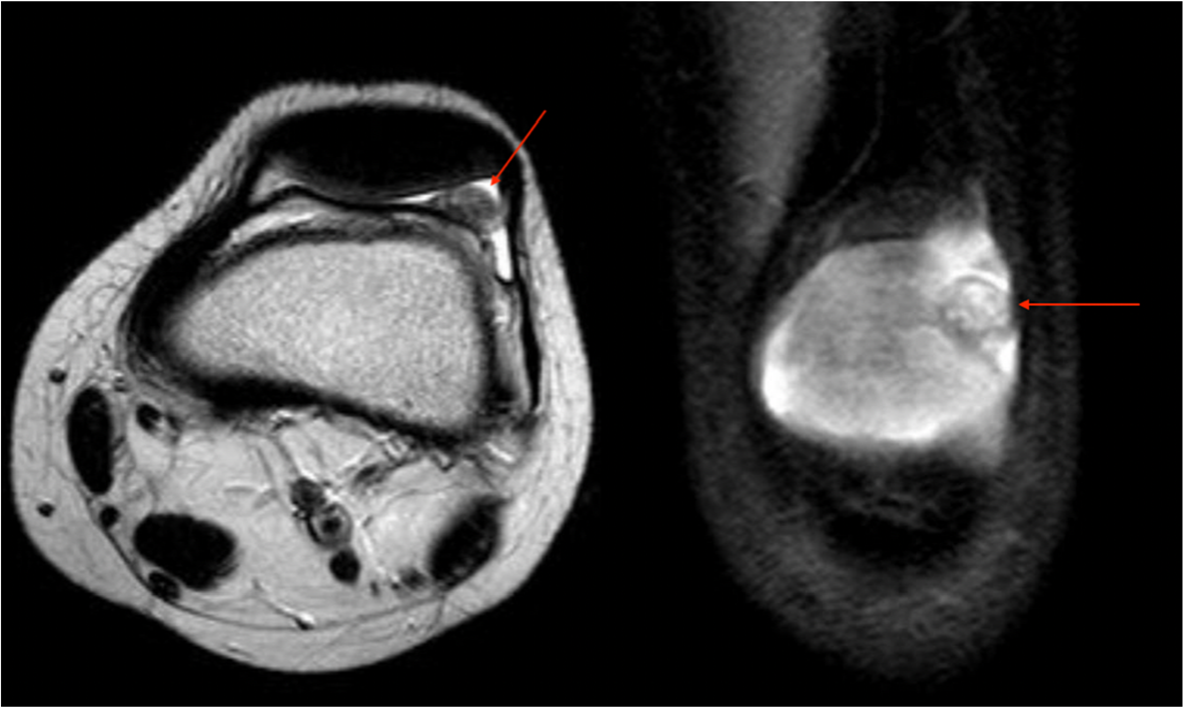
Axial T2 and coronal PD fat sat MRI scans of the left knee showing the lesion (red rows). Unfortunately, limited quality without gadolinium enhancement of the left knee as outside MR study were submitted as pre-op MRI. As well, no gradient cartilage sequences were included in the study. So, we were unable to comment about the above-mentioned sequences
